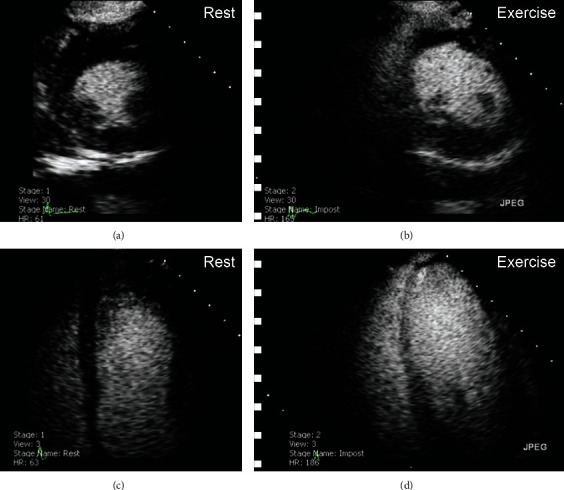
Stress echocardiogram with contrast. Parasternal mid left ventricular short axis slices (a, b) and 4-chamber views (c, d). Images (a) and (c) are taken at rest. Images (b) and (d) are taken at peak exercise, demonstrating apical ballooning.
